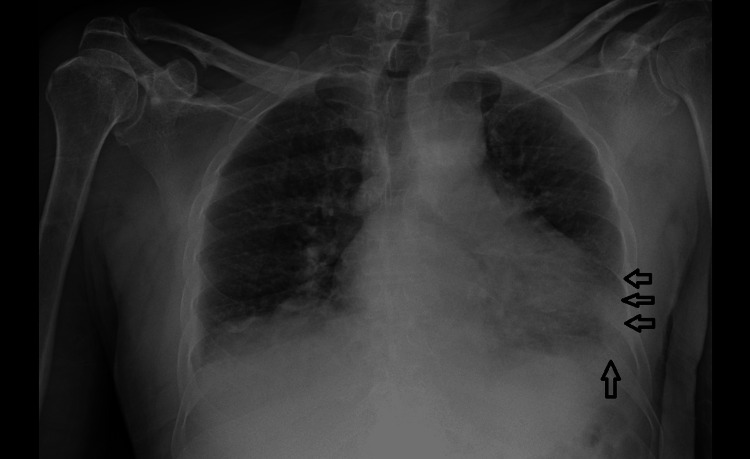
Admission chest X-ray The chest X-ray shows left lower zone consolidation with small bilateral pleural effusions
